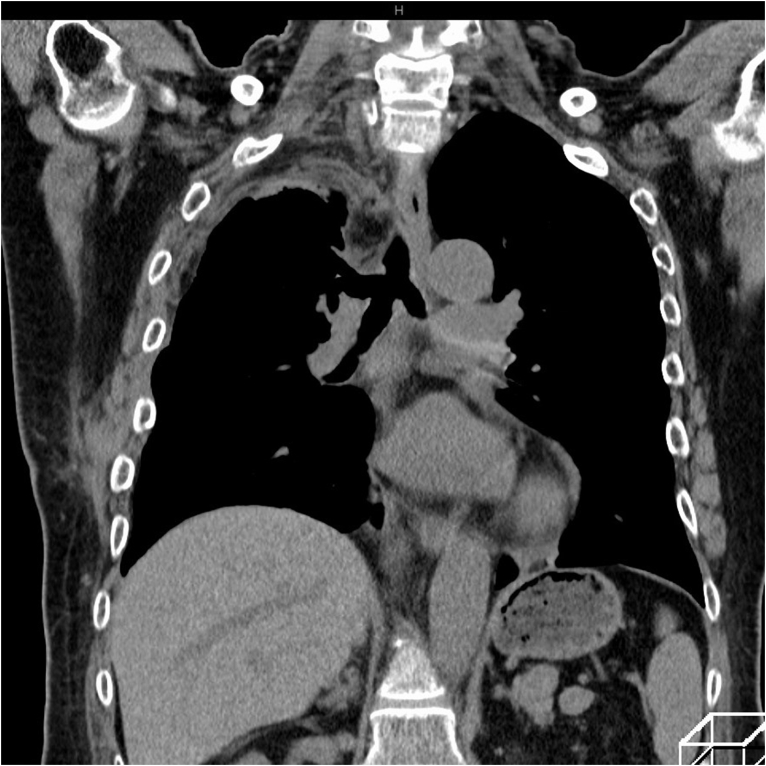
Postoperative computed tomographic scan of the chest performed 2 months postoperatively showed no stenosis at the anastomosed site. A white arrow shows latissimus dorsi flap.
